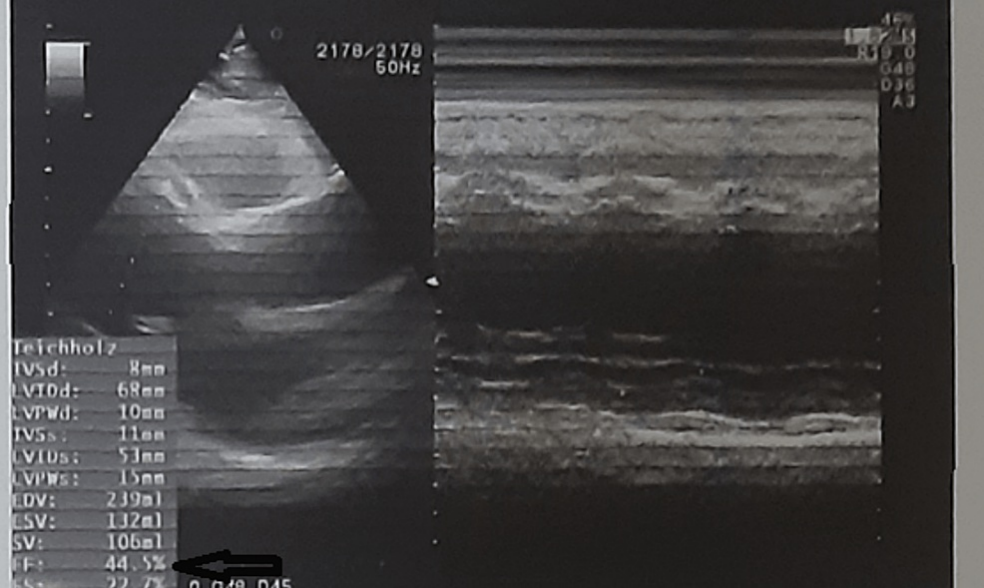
Echocardiography one week after discharge, demonstrating systolic dysfunction. Left ventricular ejection function 45%.
